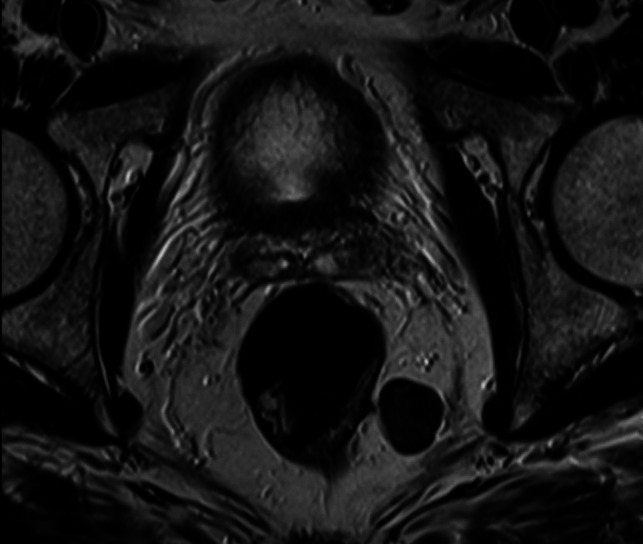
Pelvic MRI confirming the broad-based, noninfiltrative contact of the tumor with the levator ani muscle
